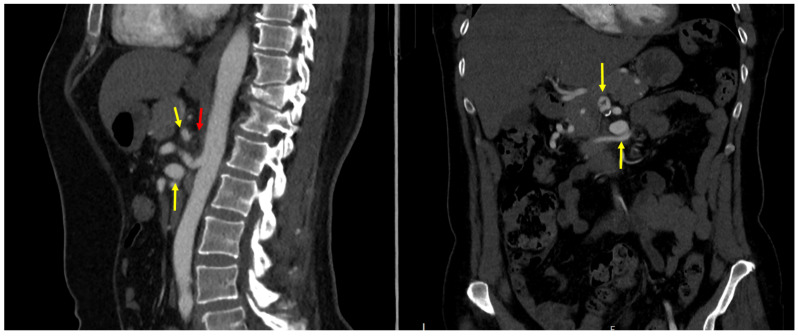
Computed tomography angiography of a VAPUS 1 A-B with a celiac axis stenosis caused by median arcuate ligament compression (red arrow) and an aneurysm of the gastroduodenal artery (upper yellow arrow) as well as the inferior pancreaticoduodenal artery (bottom yellow arrow).
